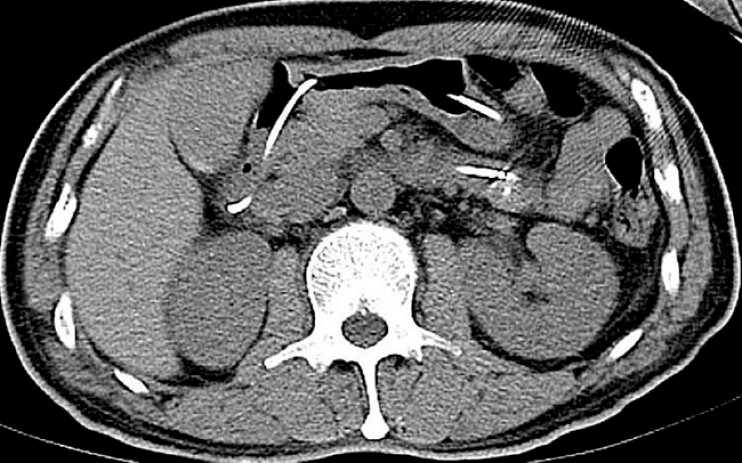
Repeat computed tomography image showing a pigtail nasobiliary drain that was placed in the duodenum to facilitate drainage, and no evidence of a residual foreign body.
